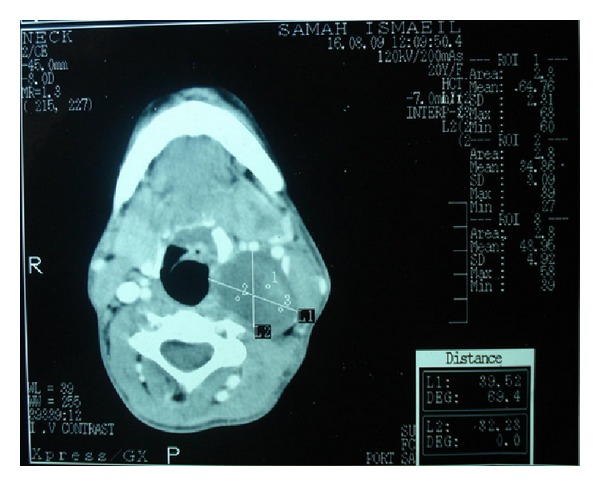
Axial view of CT showing dimensions of the tumor.
